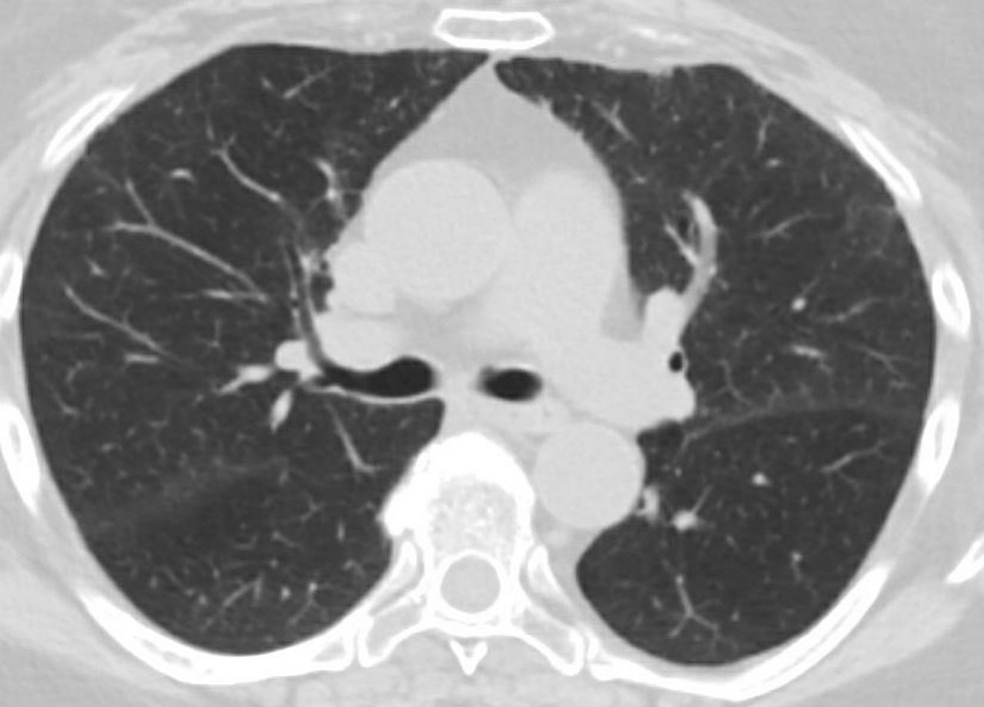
Chest CT scan showing complete clearing of pulmonary opacities after 12 weeks of treatment
